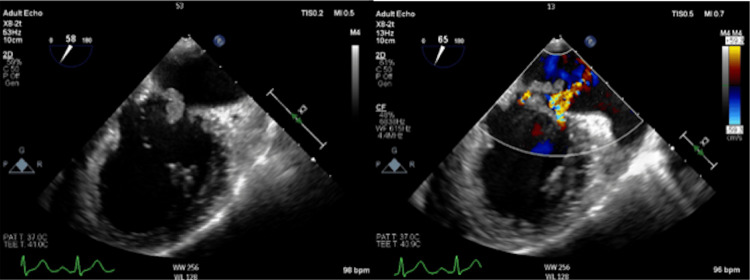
Transesophageal echocardiogram (long-axis view) reveals vegetation on the mitral valve (8–20 mm) and severe mitral regurgitation.
